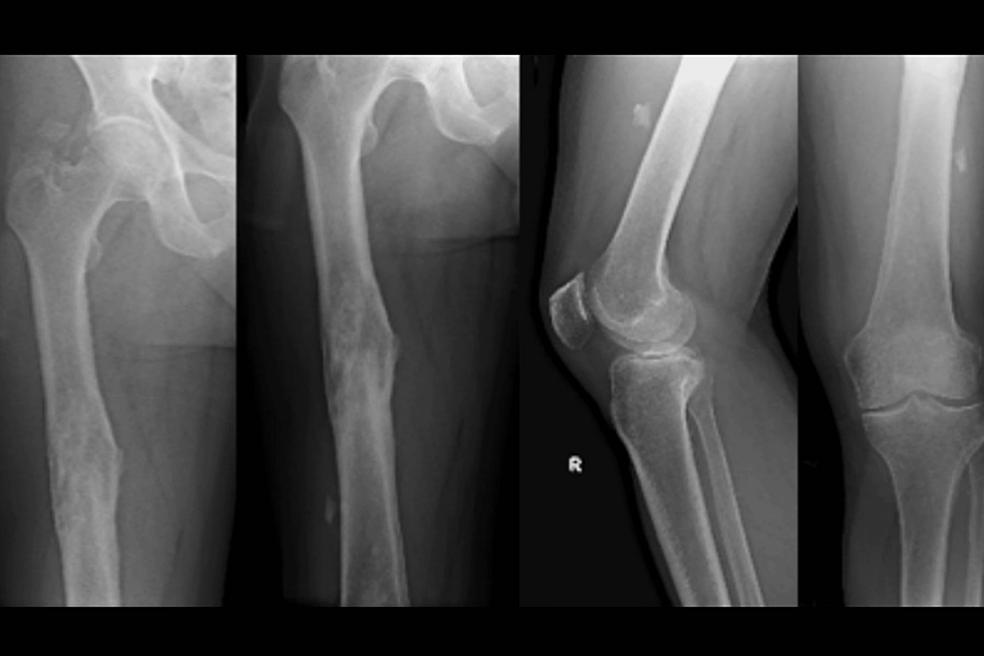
Preoperative radiographs of the right femur, and the surrounding hip and knee joints show bony changes in the femoral mid-shaft.
